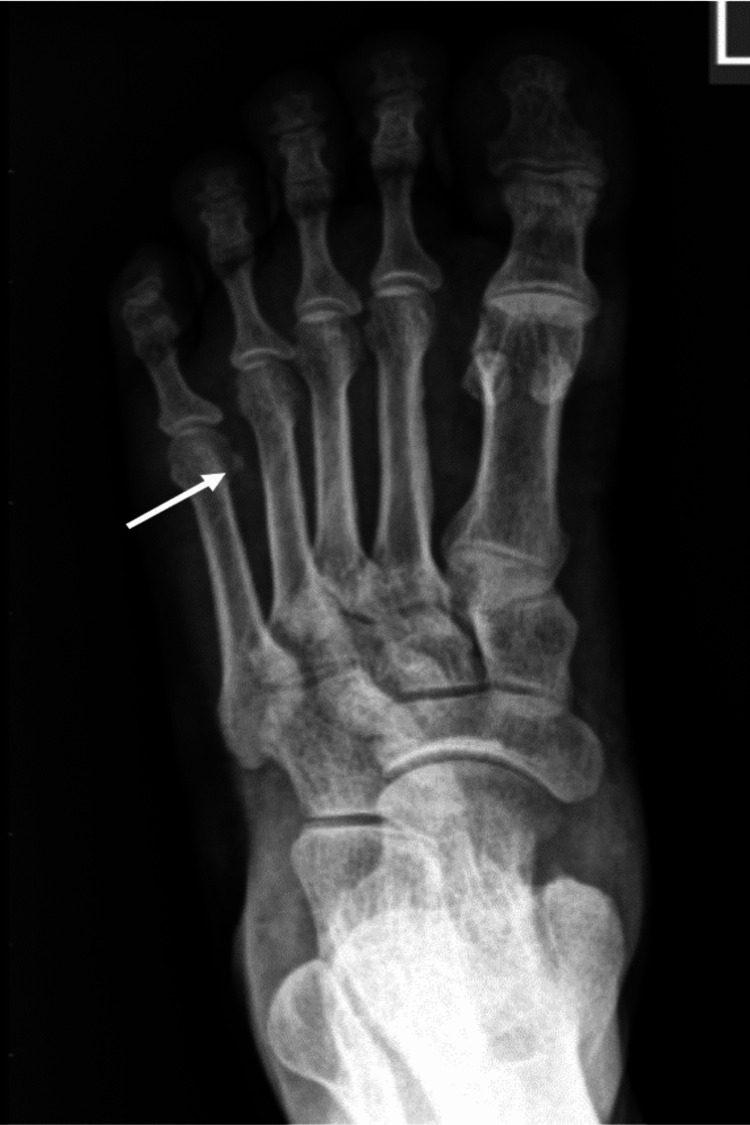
X-ray of the left foot showed chondrocalcinosis (arrow). Chondrocalcinosis appears on conventional radiograph as linear or punctate deposits (calcification) within the cartilage of the affected joint and is suggestive of pseudogout (calcium pyrophosphate deposition disease).
